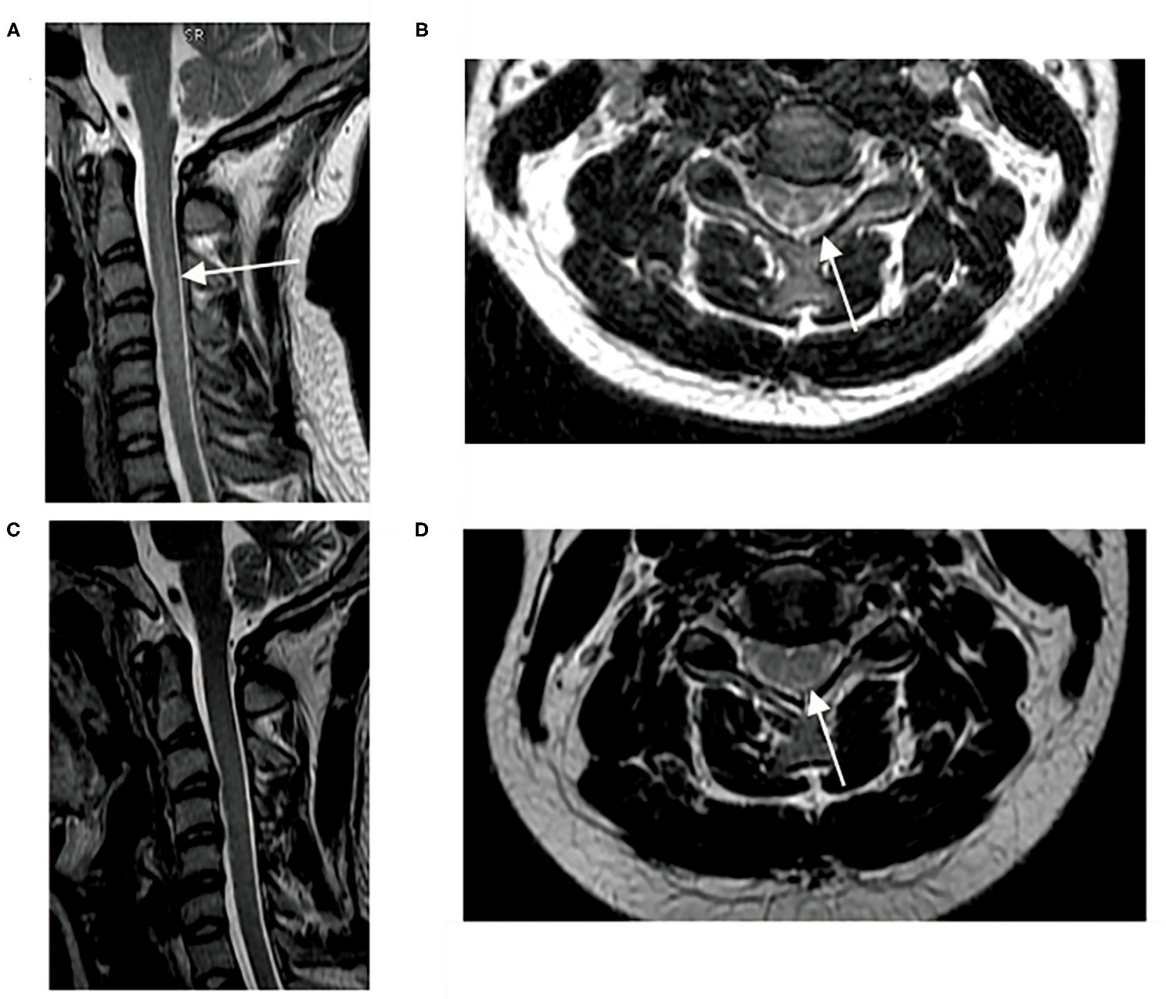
Spinal cord MRI pre- and after-treatment. (A,B) T2 hyperintensity of posterior column from C2–C6 pre-treatment; (C) Sagittal T2-hyperintensity of posterior column from C2–C6 resolved 4 months after treatment with mecobalamine and hyperbaric oxygen; (D) Axial inverted “V” T2-hyperintensity was blurred 4 months after treatment.
